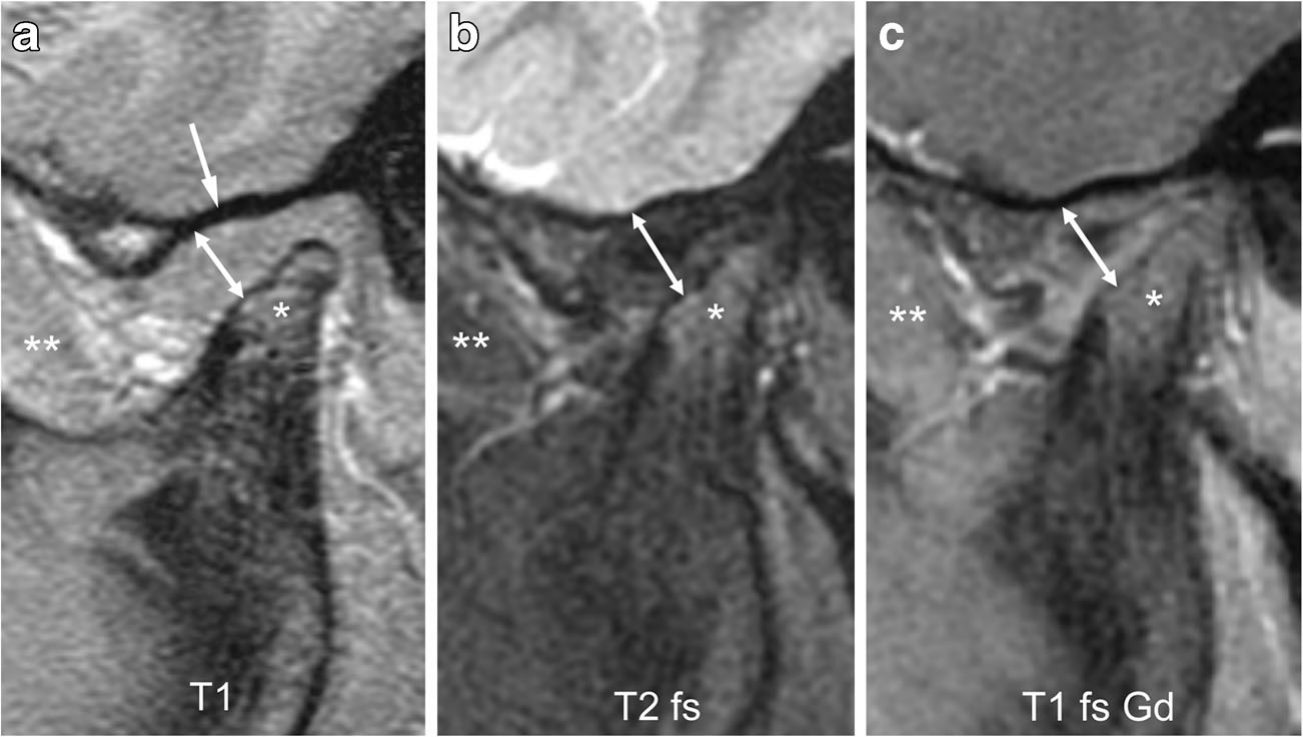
Severe synovial thickening (grade 2) but no increased joint enhancement (grade 0) on sagittal oblique images in an 8-year-old girl. a-c There is moderate flattening of the mandibular condyle (*) and mandibular fossa (arrow in a), and joint space expansion (double arrow) on T1-weighted image (TR/TE 300/4.2 ms, flip angle 80°, a), fat-saturated T2-weighted fast spin echo image (TR/TE 5,400/77 ms, b) and on postcontrast fat-saturated T1-weighted image (TR/TE 670/10 ms, c). The synovium is isointense to muscle () on all images. T1 T1-weighted, T1 fs Gd contrast-enhanced fat saturated T1-weighted, T2 fs fat saturated T2-weighted
